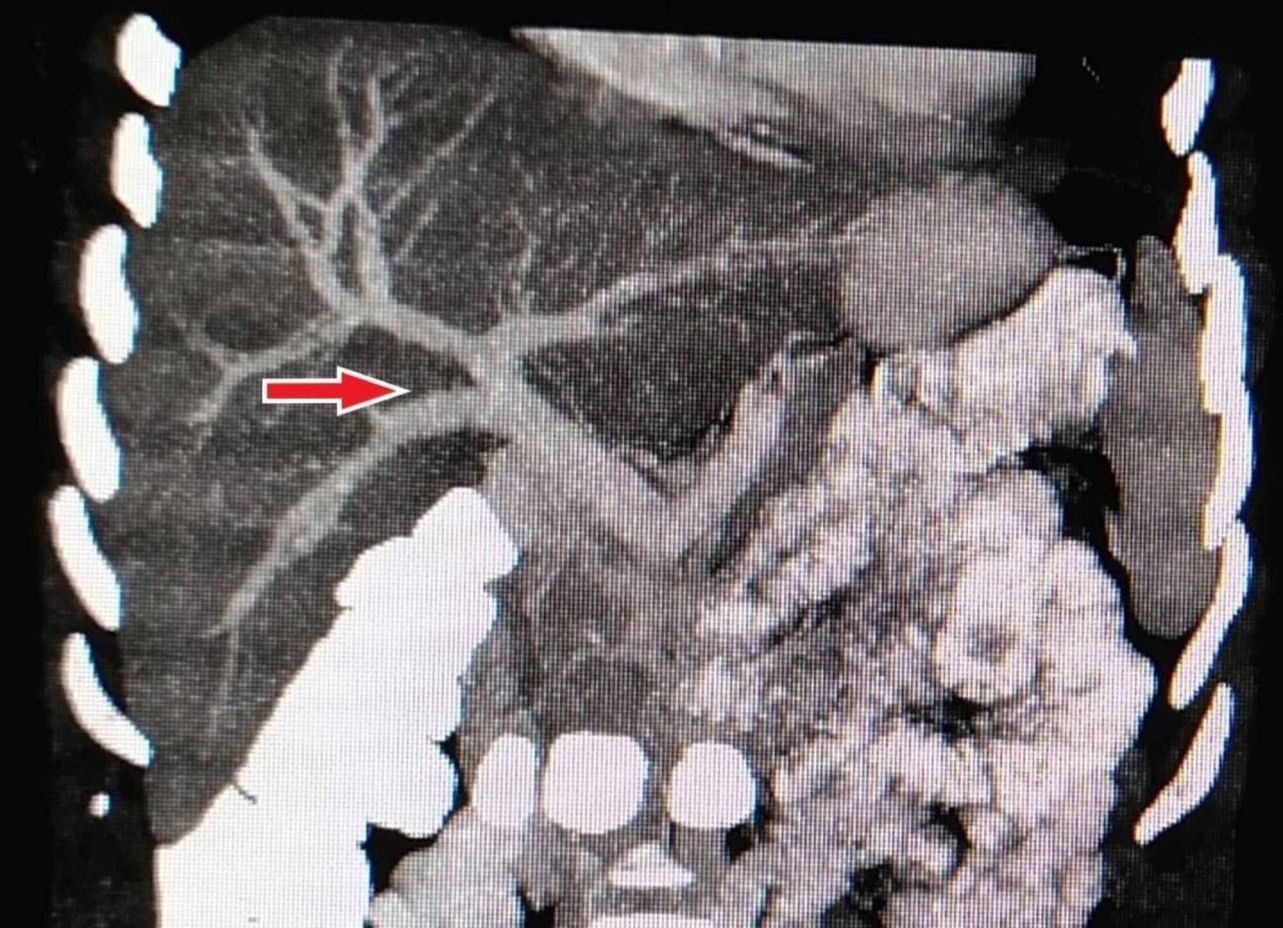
Contrast-enhanced CT MIP reconstructed coronal image showing the origin of RPPV as the first branch of the main portal vein (type 3) MIP, maximum intensity projection; RPPV, right posterior portal vein.
